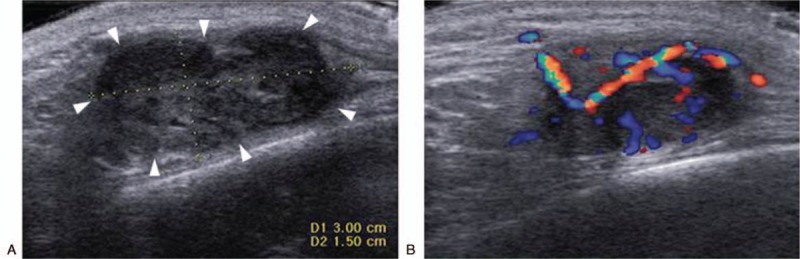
Gray-scale (A) and color Doppler (B) ultrasonographic images show a somewhat ill-demarcated, lobulated hypoechoic mass (arrowheads) located subcutaneously in the left temporal region; the extent of internal vascularity is moderate.
